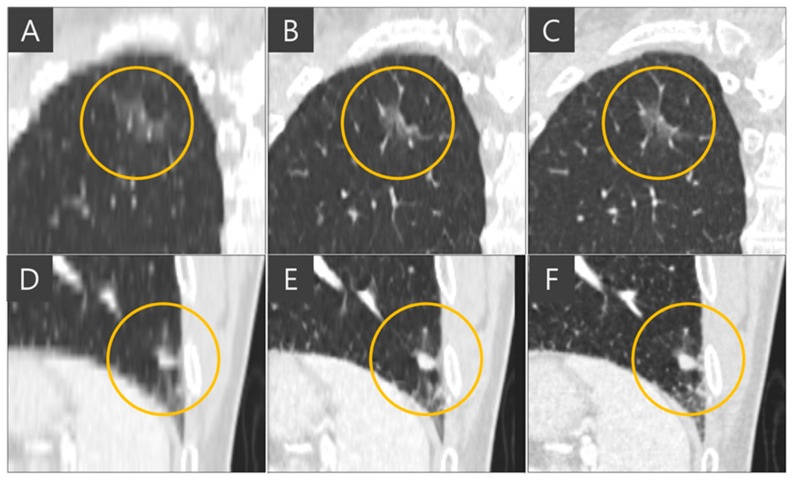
GGN circled in (A) thick-slice, (B) generated thin-slice, and (C) thin-slice CT images and solid nodule circled in (D) thick-slice (E) generated thin-slice, and (F) thin-slice CT images.
